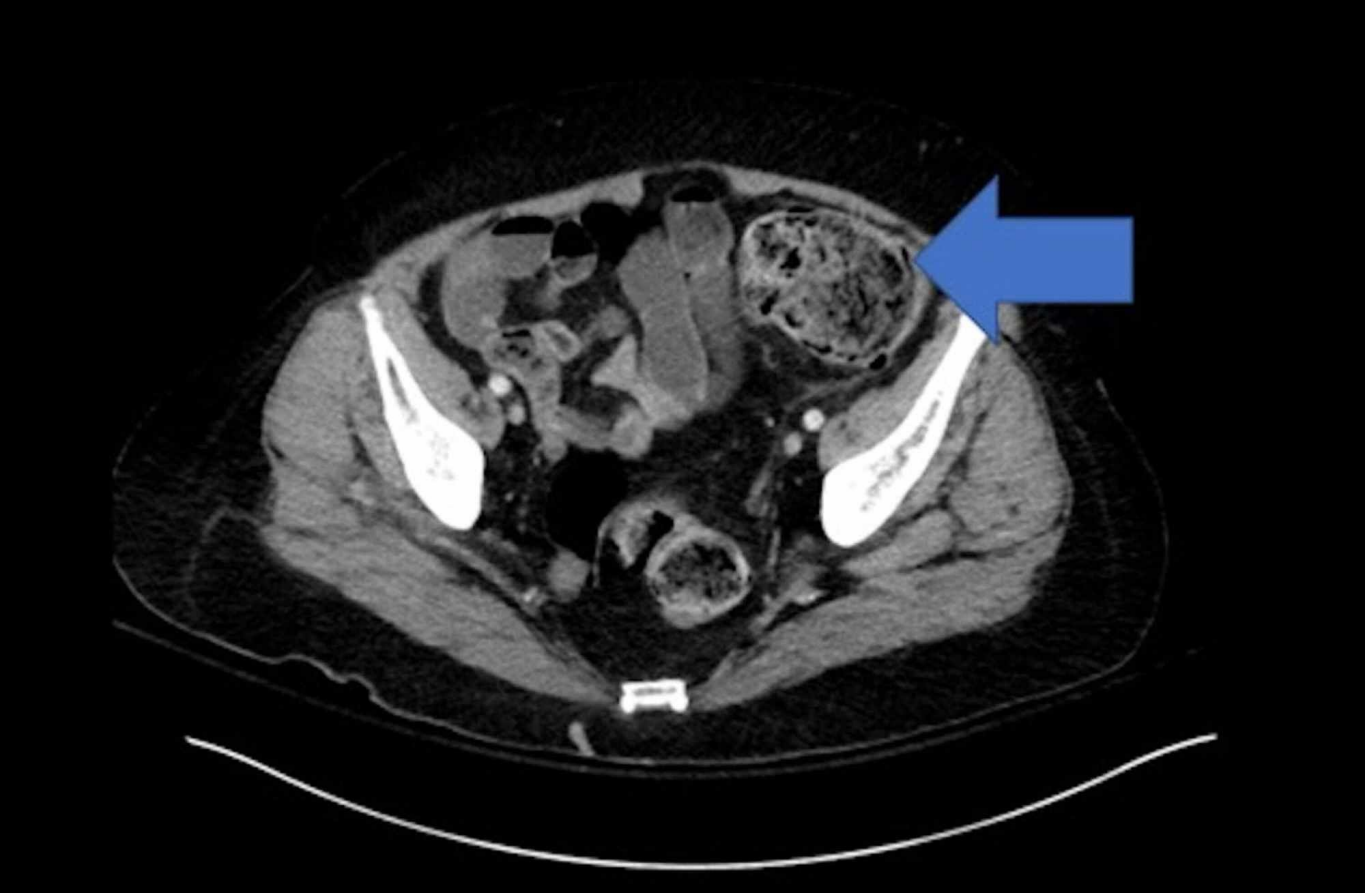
CT scan showing loaded sigmoid colon CT, computed tomography
